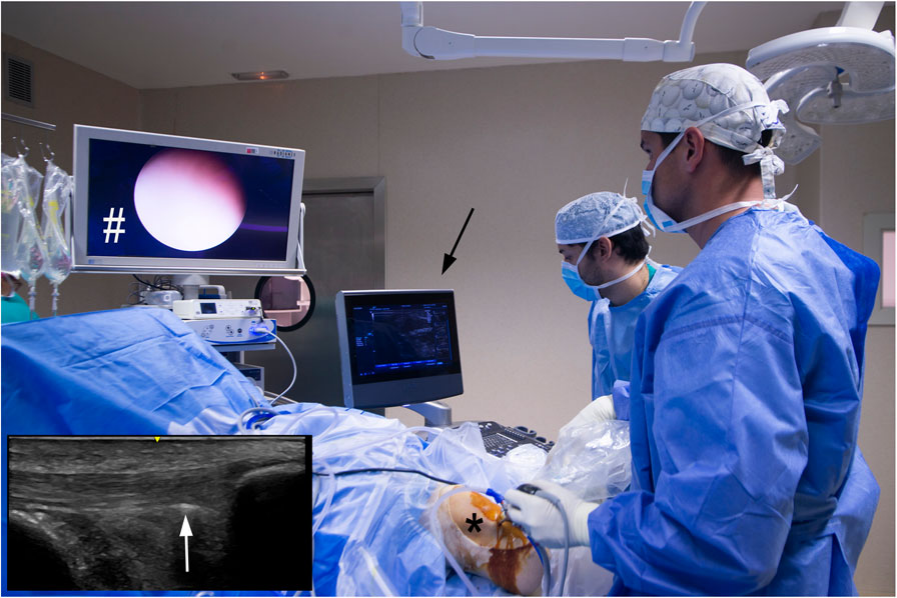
Pictures showing the US-guided (black arrow) arthroscopic (* and #) surgical set-up. Small picture shows the ultrasound view, white arrow pointing at the shaver positioned on the deep side of the proximal patellar tendon
